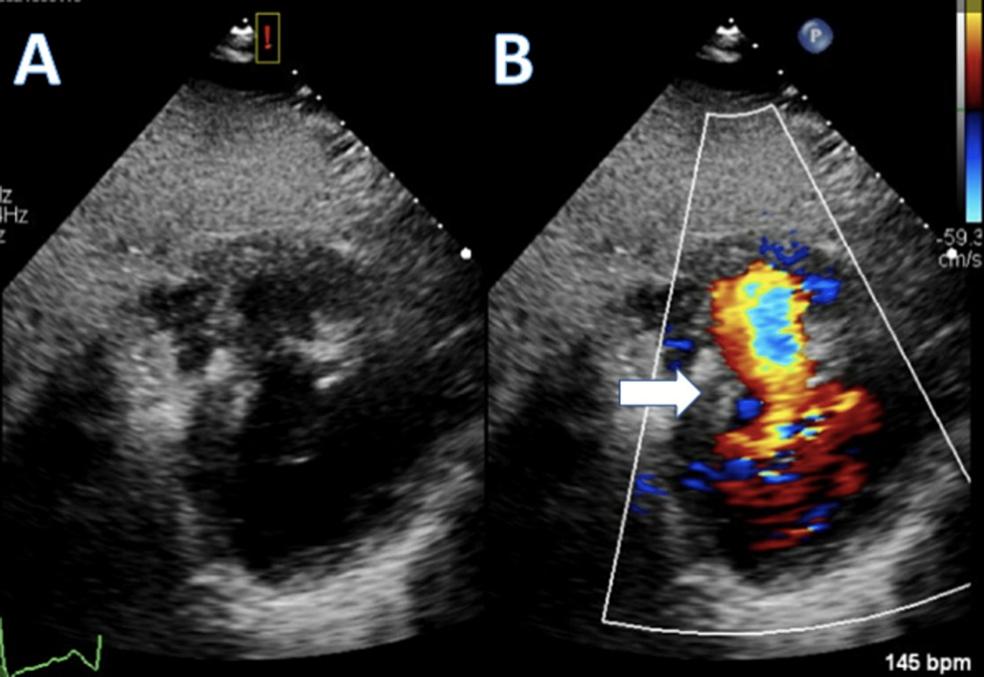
Transthoracic Echocardiogram Showing Ventricular Septal Defect A) Parasternal short axis view showing the right ventricle superior to the left ventricle; a defect is seen in the intraventricular septum. B) Color Doppler showing aberrant blood flow between the right and left ventricles through a defect in the septal wall (white arrow)
